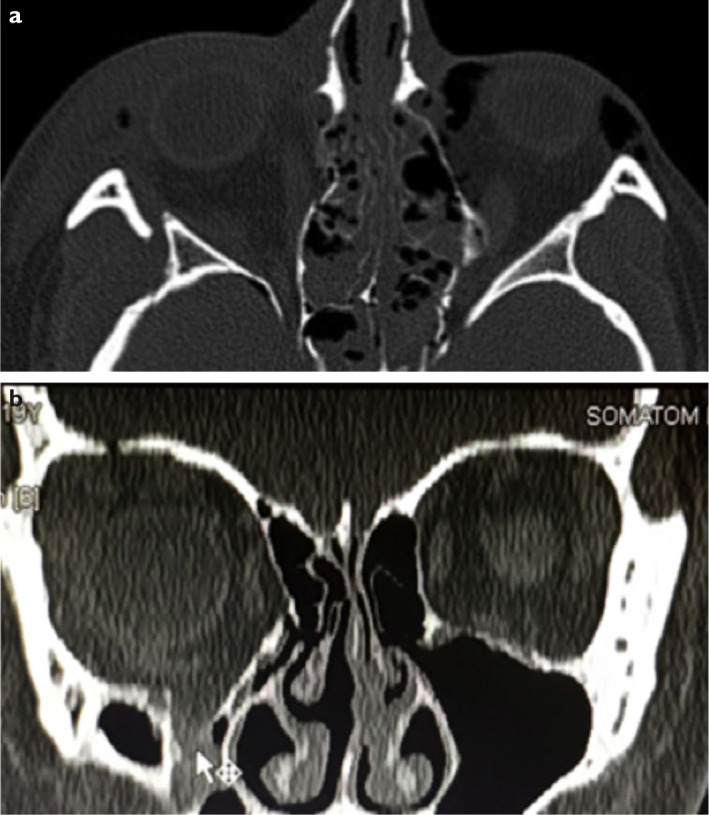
CT scans at the initial presentation showing. (a) Axial scan with lateral and medial wall fracture. (b) Coronal scan with floor and medial wall fractures.
